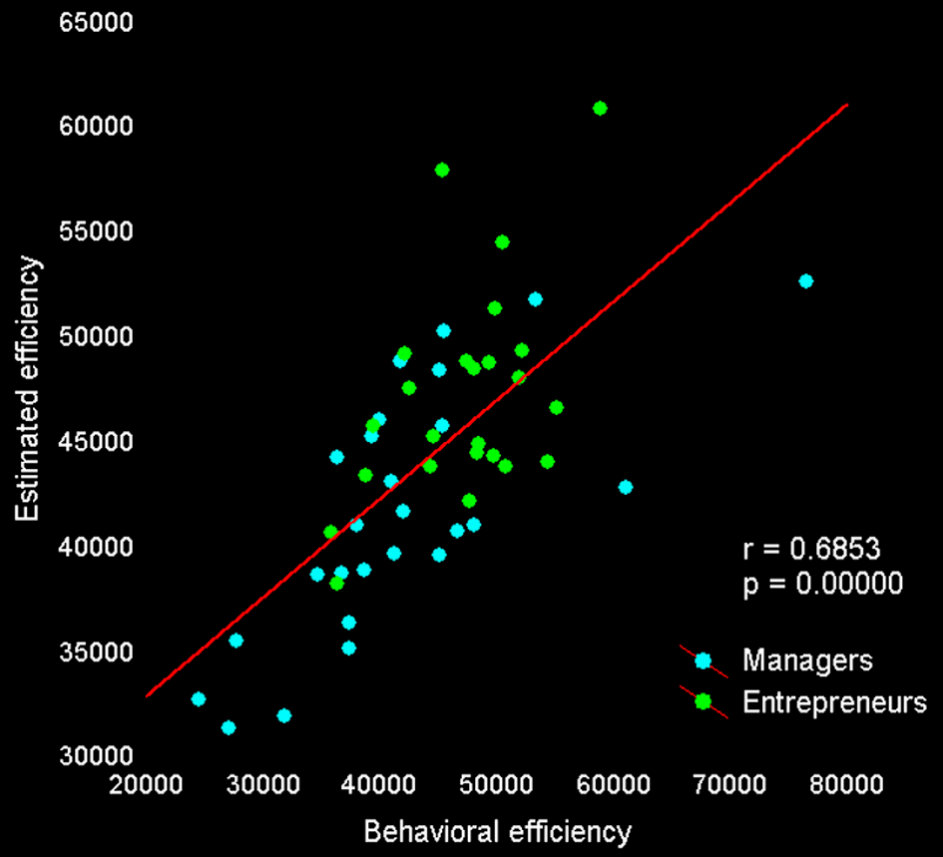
Behavioral and predicted efficiency scores. The relationship between *behavioral* decision-making efficiency (payoff divided by response time) during *f*MRI scanning and *estimated* efficiency, based on the activity of seven regions reported in previous studies and showing a significant difference between exploitative and explorative choice [vmPFC, bilateral FPC, and IPS from Daw et al. (2006); right FPC by Boorman et al. (2009); dorsal sector of anterior cingulate cortex by Kolling et al. (2012)].
